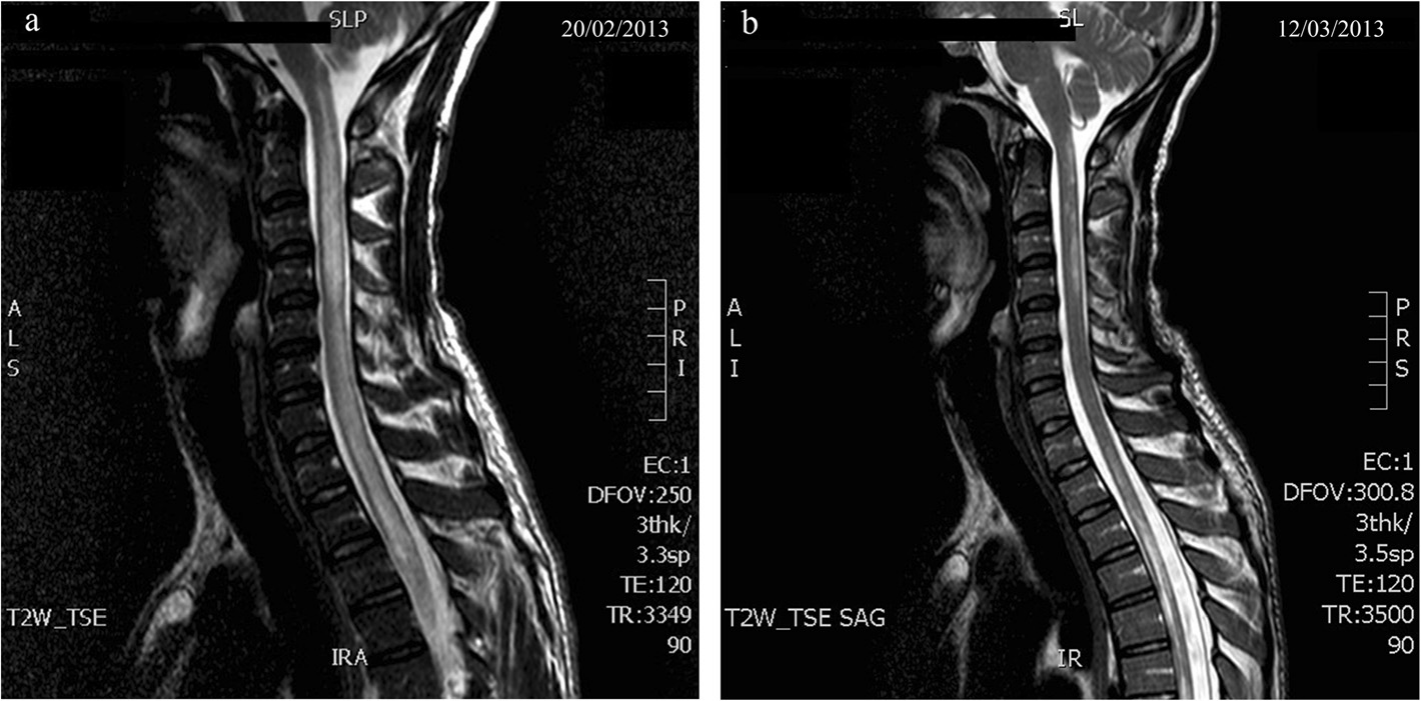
Spinal cord MRI images before (a) and after (b) immunosuppressive treatment. a: Sagittal spinal cord MRI image demonstrating signs of longitudinally extensive transverse myelitis that affects the entire spinal cord and spreads upwards to the lower regions of the medulla oblongata. b: Sagittal spinal cord MRI image which shows a clear reduction of the areas with altered signal intensity.
